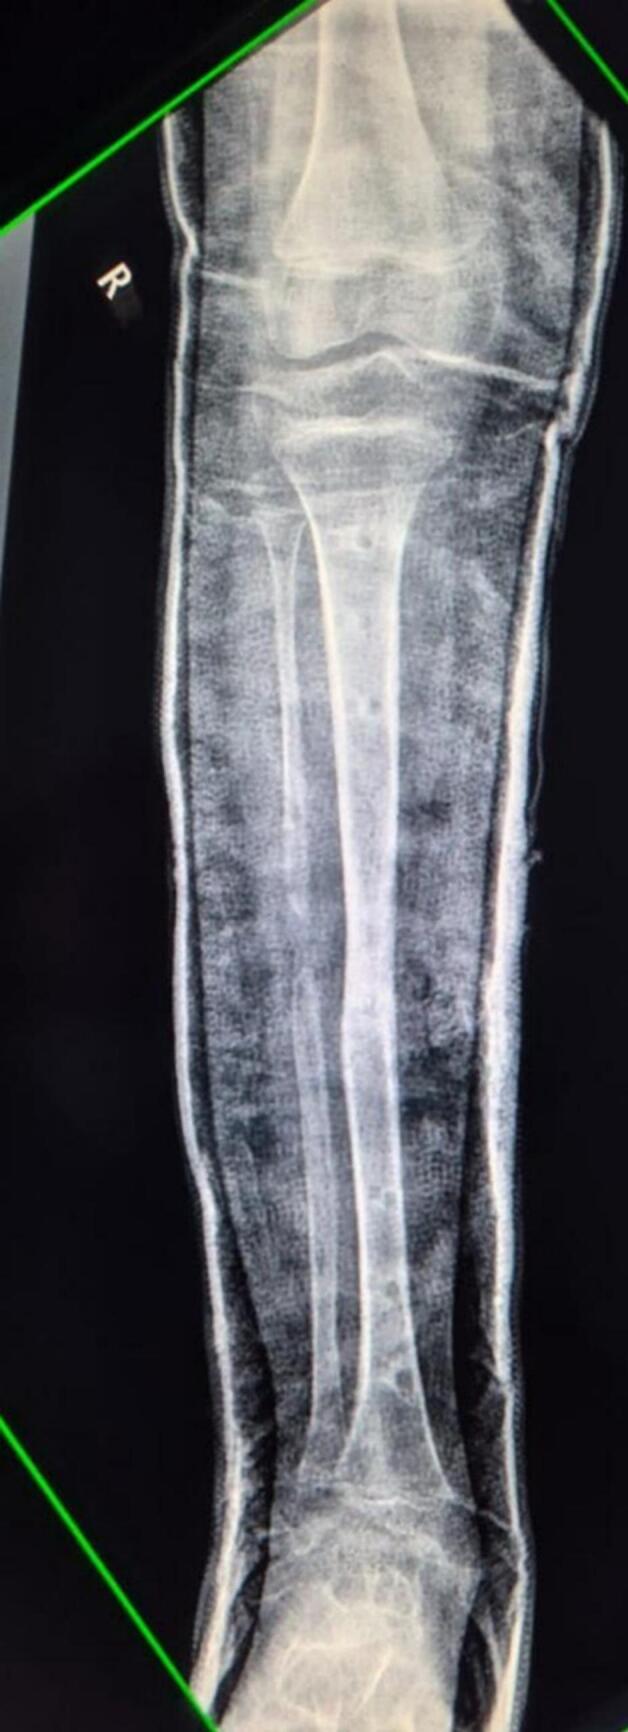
Xray Right leg (AP view) with above knee cast insitu for stabilization post LRS fixator removal.
